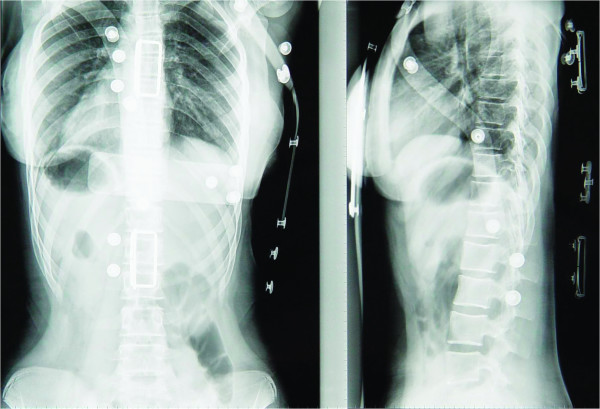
Standing (brace worn) posterior-anterior and lateral radiographs 24 months after the initial application of the brace (Visit 3).
